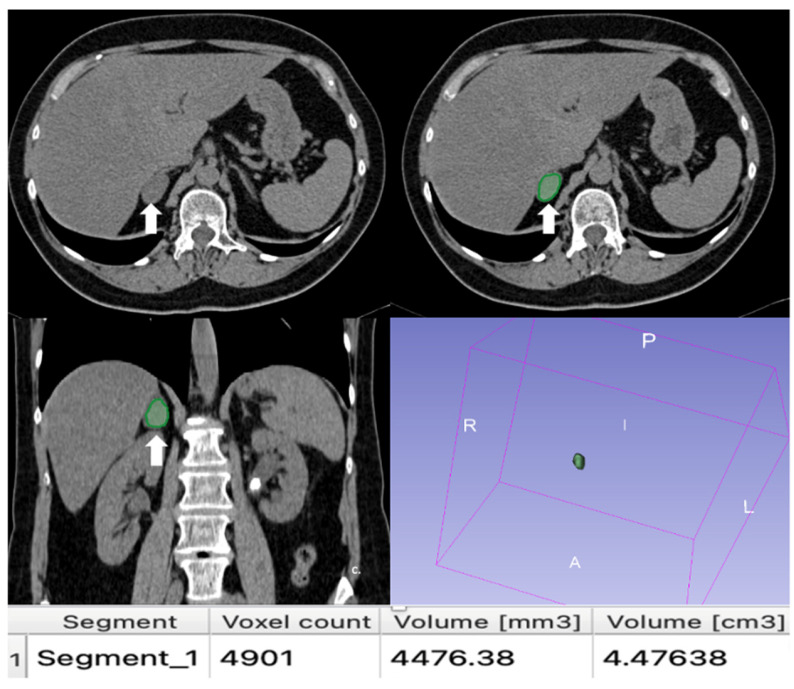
An example of segmentation of an LPA in the right adrenal gland (arrow) in an unenhanced CT phase using 3D Slicer software (version 4.11). The volume of interest (VOI) of the LPA was manually drawn covering the whole lesion.
